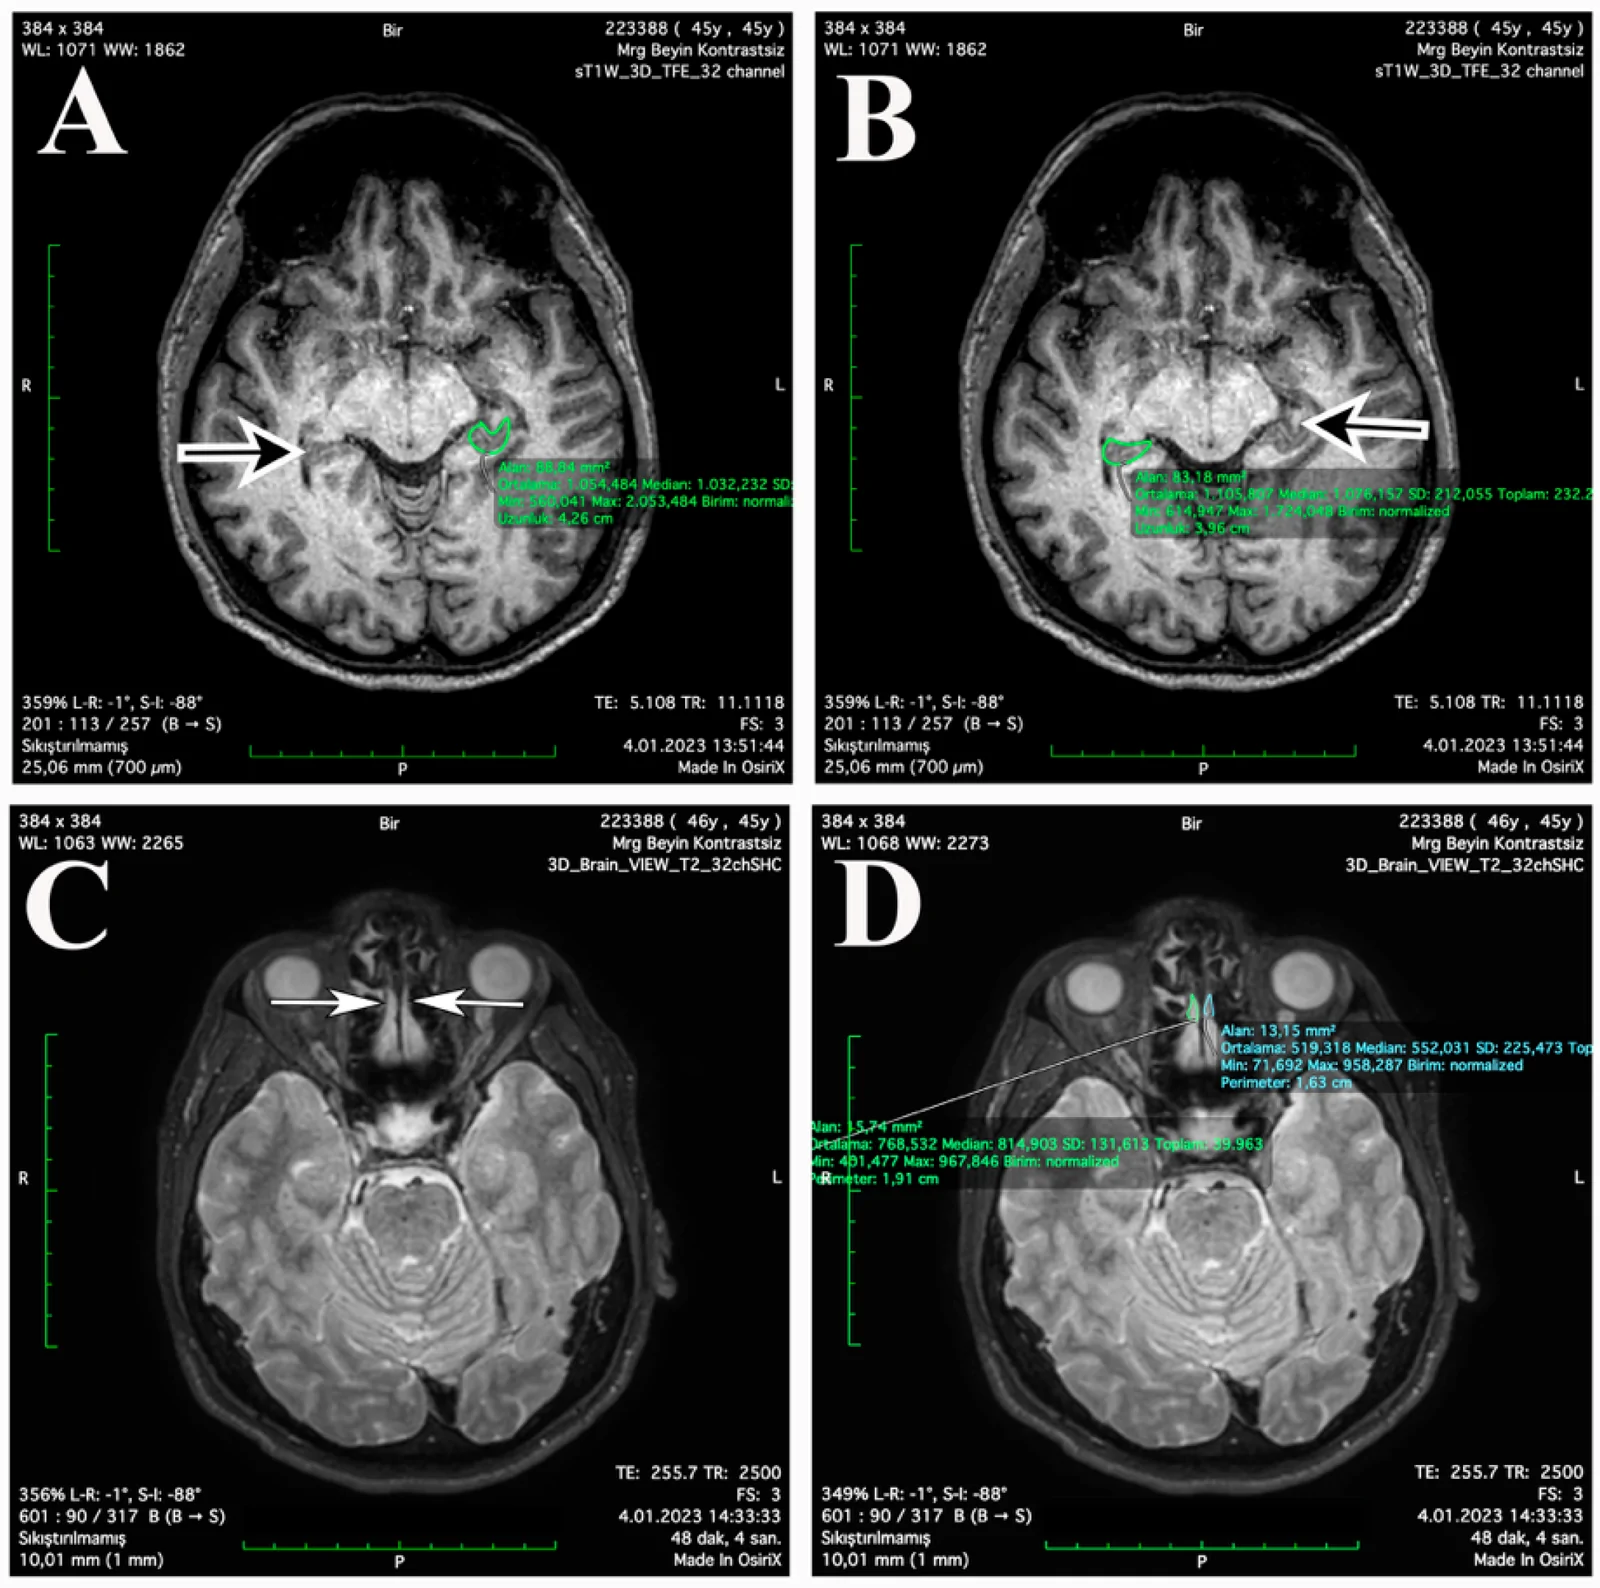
The planimetric analysis of the olfactory bulb and hippocampus regions in the MR images obtained from the sT1W_3D_TFE and 3D_Brain_VIEW_T2 sequences is presented. (A,B) Measurements in Osirix Lite of hippocampal and (C,D) olfactory bulb regions. Black arrow: hippocampus; white arrow: olfactory bulb.
